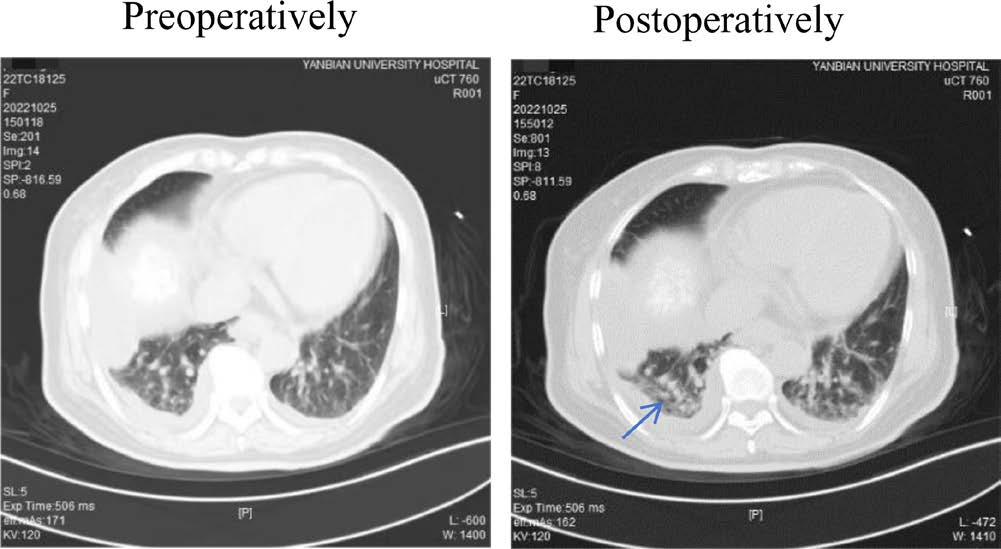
CT images before and after surgery.
